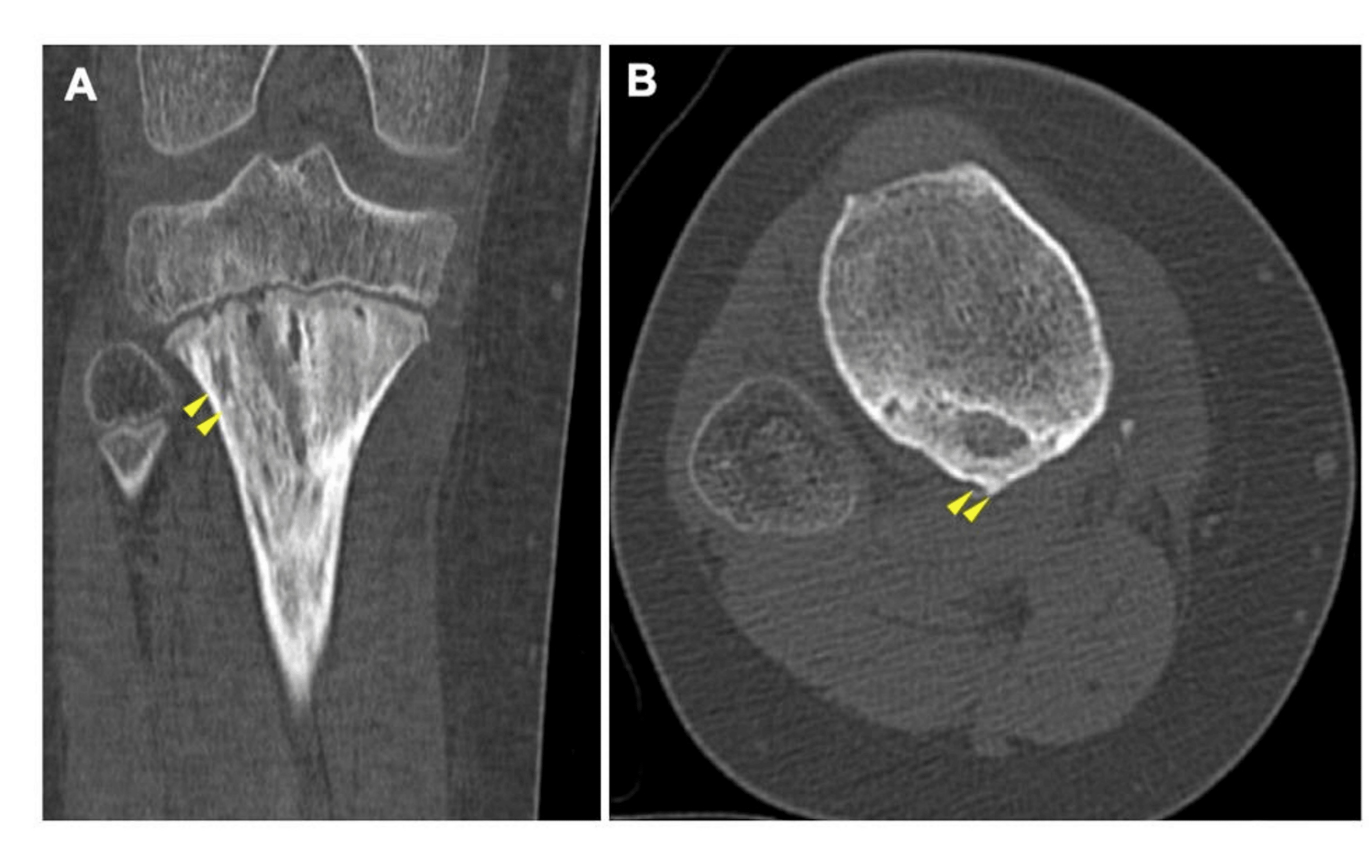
Computed tomography images of the right knee. (A) Coronal view and (B) axial view. Computed tomography images show bone destruction with some calcification in the proximal tibia (yellow arrow).
